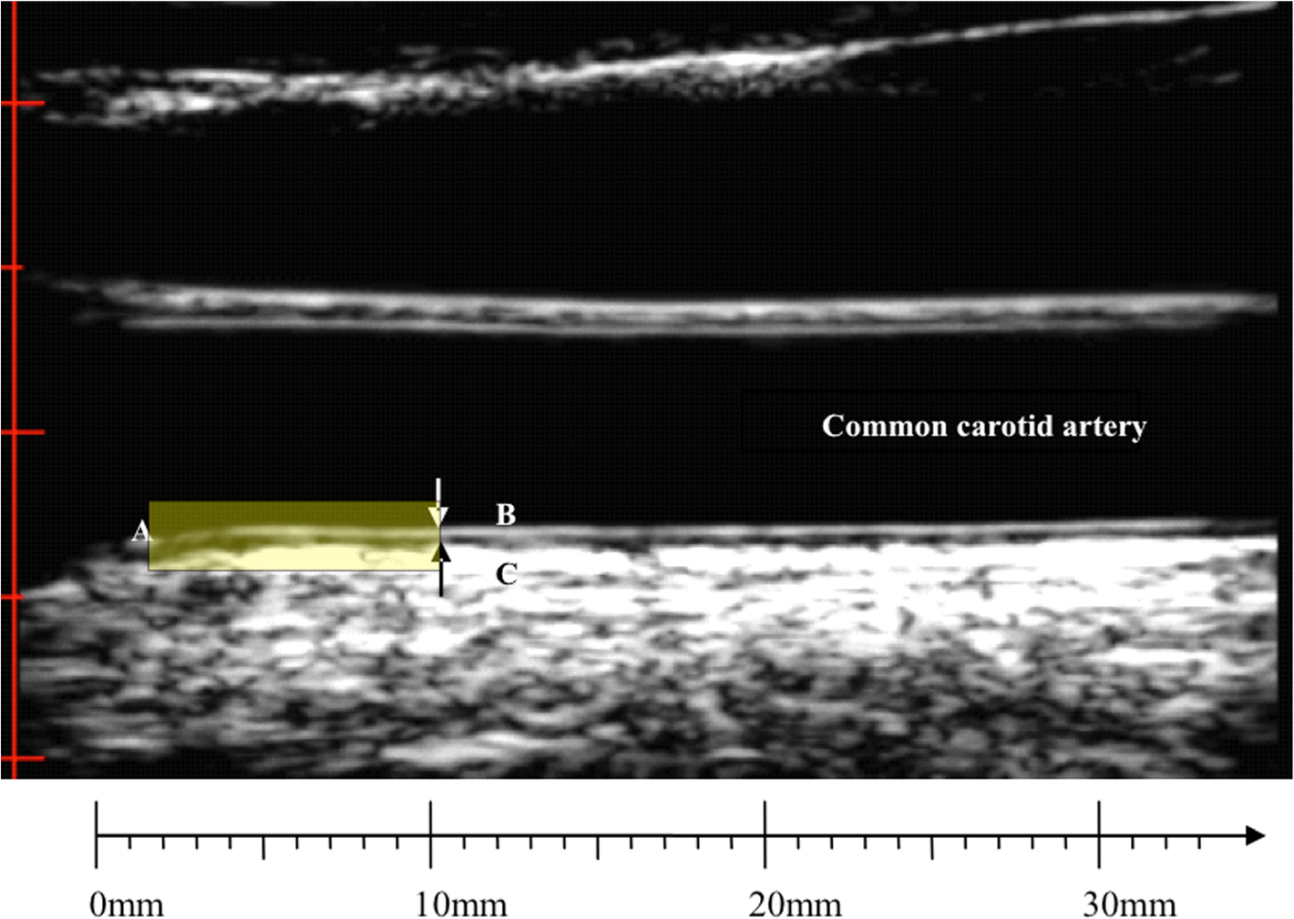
B-mode ultrasound image of the common carotid artery (CCA): a border of carotid bulb widening (0 mm), (b) CCA far wall lumen-intima interface, (c) media-adventitia interface. Definitions of the CCA segment for measurement differ depending on study and convention, but are typically measured in the vicinity 0–10 mm proximal to the border of the bulb widening (a) (measurement area highlighted by *yellow box*). CCA IMT is taken as the distance between (b) and (c)
